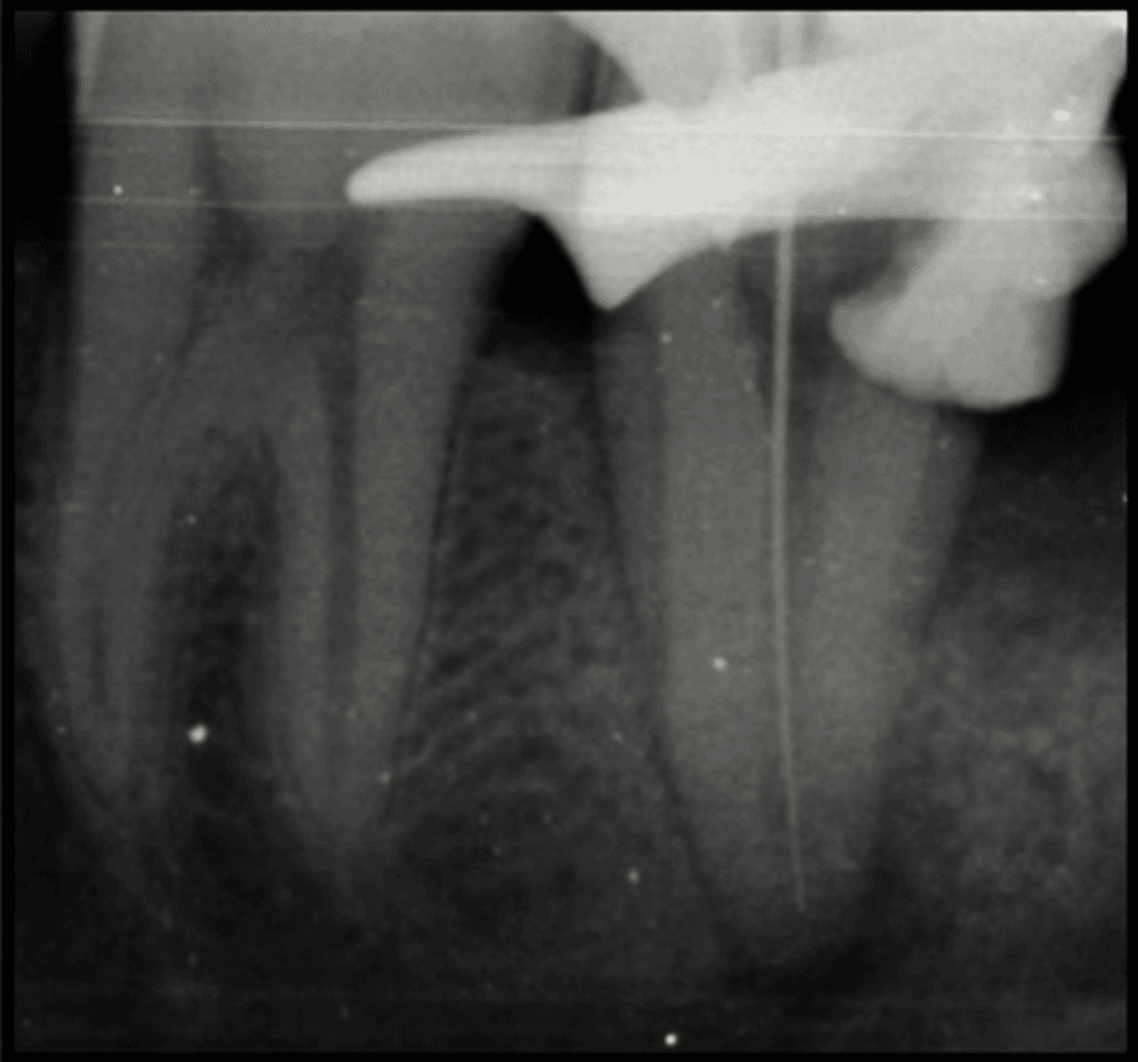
Intraoral periapical radiograph of working length determination with 47 The intraoral periapical radiograph shows a working length determination of 47 using a 15K file
